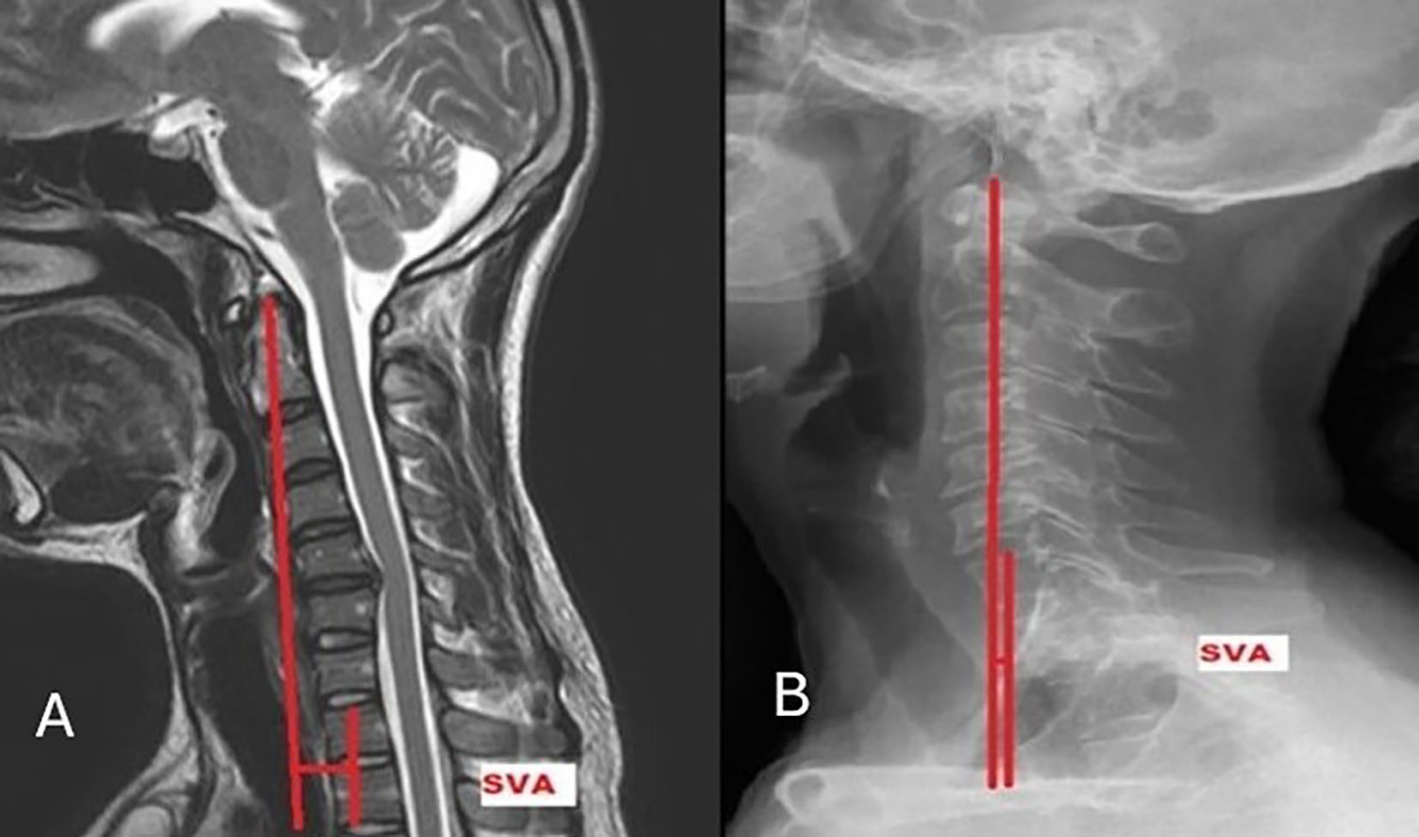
Measurement of C2-C7 sagittal vertical axis on MRI (A) and radiograph (B).
